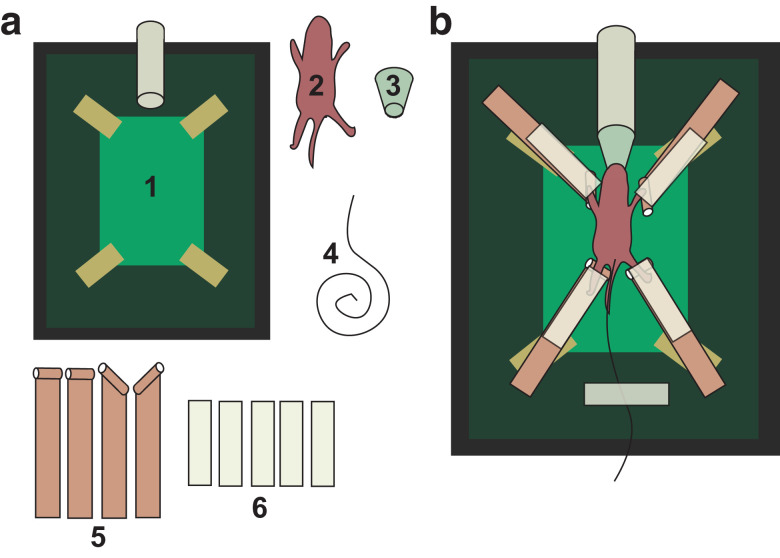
Setup for assessing neonatal cardiac function and structure using high-resolution *in vivo* ultrasound imaging. (a) Adult setup for ultrasound imaging. A standard ultrasound table (*1*) was used. The neonatal pup (*2*) was placed on the table, which was adapted using a nose cone and adapter (*3*), a neonatal rectal thermometer (*4*), conductive copper tape (*5*) and regular tape (*6*) as shown on panel b.
